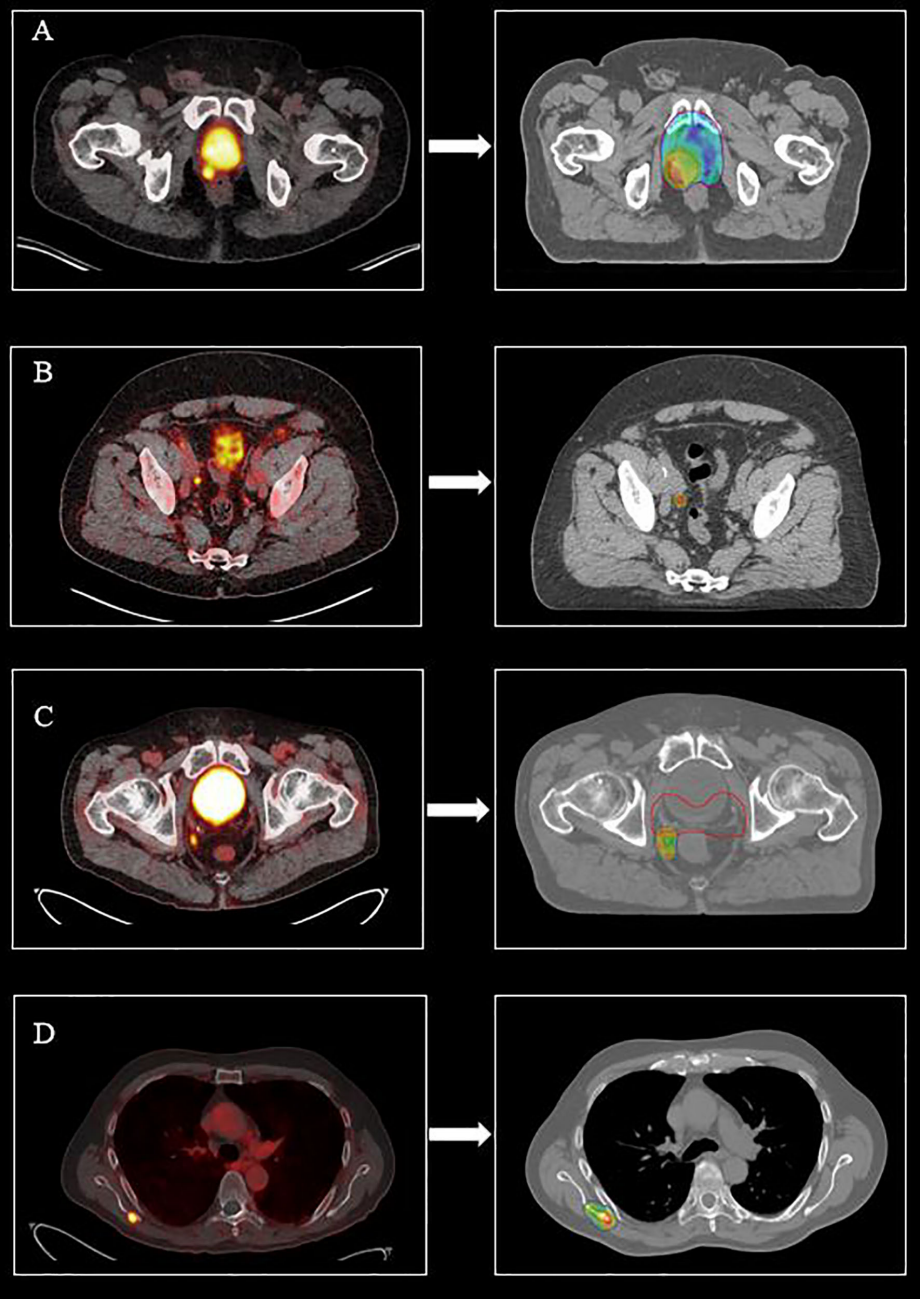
^68^Ga-PSMA PET/CT restaging findings and the corresponding salvage radiotherapy treatments (color wash isodose line 95%) in patients with biochemical recurrent prostate cancer after radical prostatectomy. (A)^68^Ga-PSMA PET/CT revealing a prostate bed recurrence located close to the bladder neck (left). Prostate bed radiotherapy (64 Gy/32 fx) planned with a simultaneous integrated boost (70.4 Gy/32 fx) (right) to the PET/CT positive lesion. (B)^68^Ga PSMA PET/CT revealing a millimetric solitary right external iliac node (left). Salvage stereotactic body radiation therapy planned on the PSMA avid node (30 Gy/3 fx) (right). (C)^68^Ga PSMA PET/CT revealing a perirectal oligorecurrent nodal relapse after radical prostatectomy and salvage prostate bed radiotherapy (left). Stereotactic body radiation therapy planned on the PSMA avid node (35 Gy/5 fx). Prostate bed PTV is shown in red (right). (D)^68^Ga PSMA PET/CT revealing an oligometastatic bone metastasis located at the right scapula (left). Stereotactic body radiation therapy planned to the PET/CT positive bone lesion (30 Gy/3 fx).
